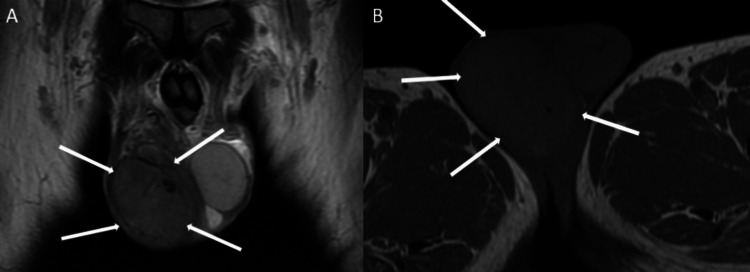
A: Coronal T2-weighted MRI section of the scrotum; the right testis is showing a hypointense mass completely occupying the testis. B: Axial T1-weighted MRI section of the scrotum; the right testis is showing an iso-intense mass completely occupying it.
